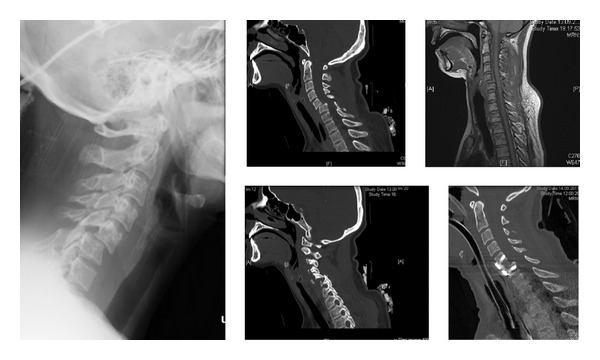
Conventional X-rays on admission showing fracture dislocation C4/5 (left); preoperative CT scan showing unilateral facet dislocation (middle); MRI after closed reduction (upper right); CT scan after anterior decompression and stabilization (lower right).
